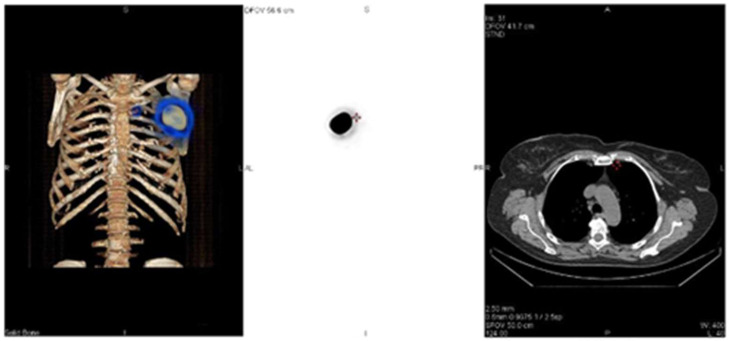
Detection by SPECT-CT of one of the two SLNs located behind the left chondrocostal joint II (bone reconstruction, nuclear medicine, and CT images); the intense area of fixation corresponds to the peritumorally injected radioactivity.
